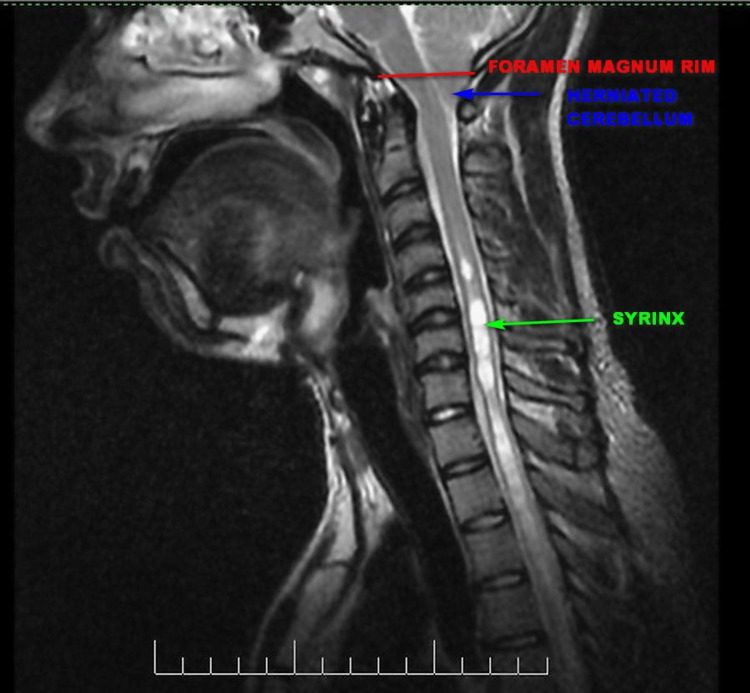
T2 weighted sagittal MRI scan done prior to the first surgery MRI: magnetic resonance imaging
